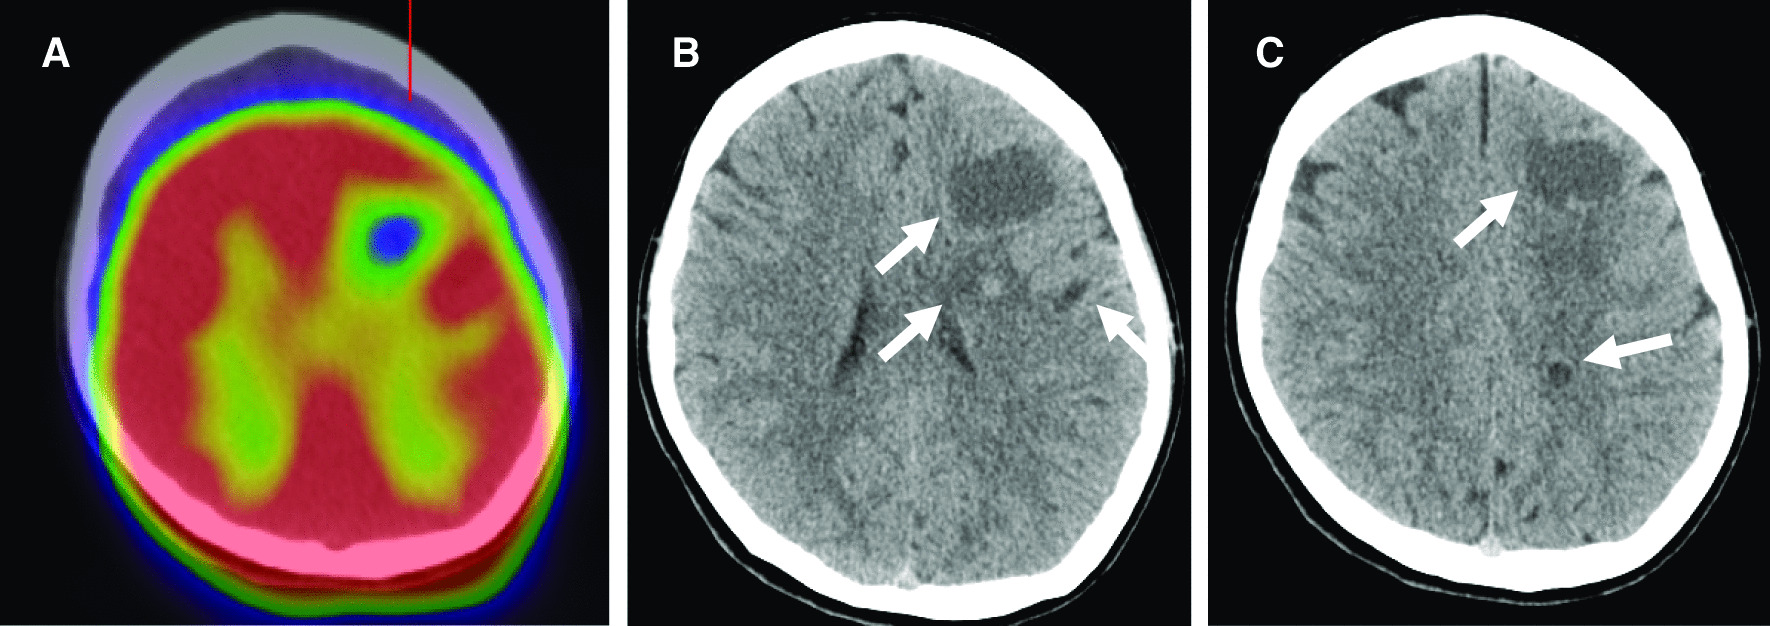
A PET revealed intracranial cold spots. B, C Brain CT scans showing cystic lesions without calcification in the parenchyma of the left hemisphere (arrows)
